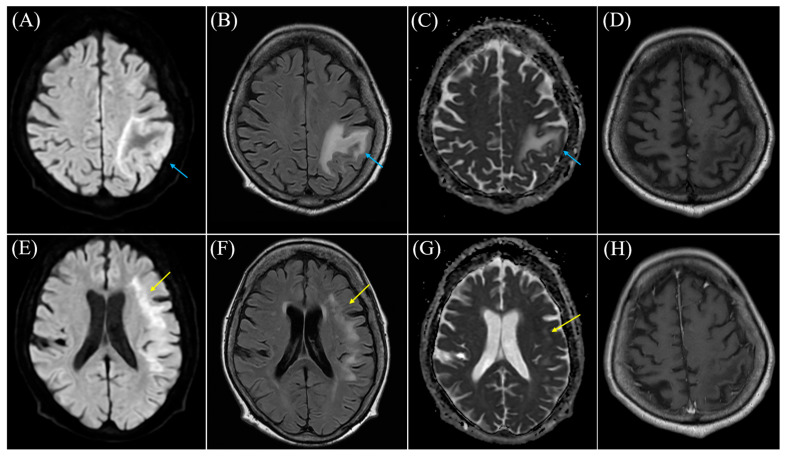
The brain magnetic resonance imaging (MRI) results obtained 3 months prior to death. (A,E) Diffusion-weighted imaging (DWI). (B,F) T2-Fluid attenuated inversion recovery (T2-FLAIR) images. (C,G) Apparent diffusion coefficient (ADC) maps. (D) T1-weighted imaging. (H) Contrast-enhanced T1-weigted imaging. DWI and T2-FLAIR images revealed a mass-like hyperintense lesion in the left parietal lobe (blue arrow), along with band-like hyperintensities extending from the frontal to the temporal lobes and involving subcortical U-fibers and deep white matter (yellow arrow). The corresponding ADC maps of these DWI hyperintense regions showed high values. The patient was undergoing treatment for malignant lymphoma, and the parietal-lobe MRI abnormalities appeared mass-like, necessitating exclusion of lymphoma metastasis or intravascular lymphoma. Cerebrospinal fluid (CSF) analysis revealed no pleocytosis or elevated protein levels, and cytology showed no abnormalities. Furthermore, there was no enhancement on contrast-enhanced MRI, supporting a low likelihood of malignant lymphoma. While a brain biopsy would have definitively excluded lymphoma, it was deferred as the patient met the criteria for ‘definite progressive multifocal leukoencephalopathy (PML)’ per the American Academy of Neurology consensus statement [1]. This diagnosis was firmly supported by the combination of progressive clinical deficits, the detection of JC virus DNA in the CSF (221 copies/mL), and the hallmark radiological finding of a massive lesion with a conspicuous absence of mass effect. Both obinutuzumab and bendamustine have been reported to be associated with a risk of PML [2,3]. Nonetheless, it is notable that PML typically shows no contrast enhancement on MRI in classic cases, but contrast enhancement often appears during immune reconstitution inflammatory syndrome (IRIS), reflecting inflammatory response [4,5,6,7,8].
